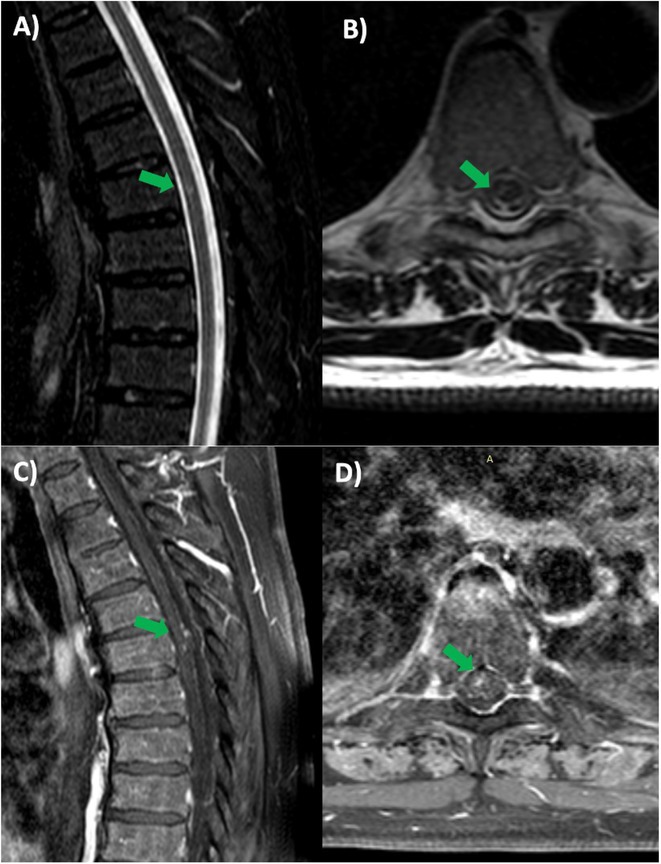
FIGURE 3 Lesion at T6 level on thoracic spinal cord MRI at first admission A. STIR, B. T2WI, C and D. T1WI with fat suppression after intravenous administration of contrast agent
